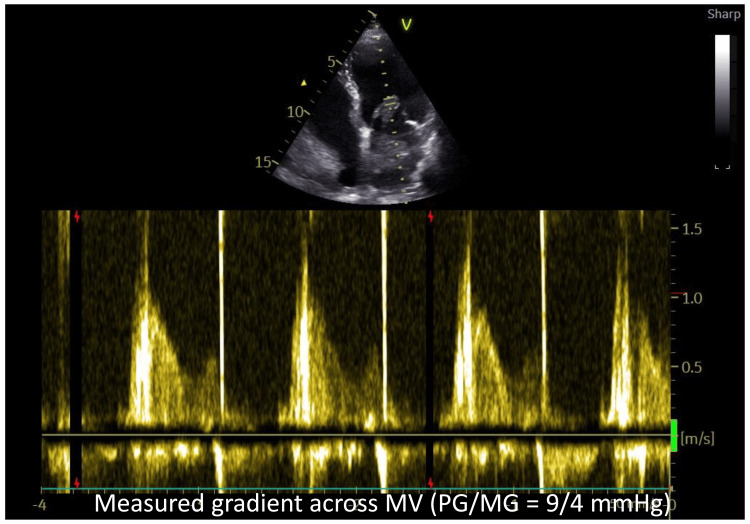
Continuous-wave (CW) Doppler interrogation across the mitral valve The CW Doppler tracing across MV demonstrates a peak gradient of 9 mmHg and mean gradient of 4 mmHg, indicating hemodynamically non-significant obstruction at rest despite the large tumor size.
